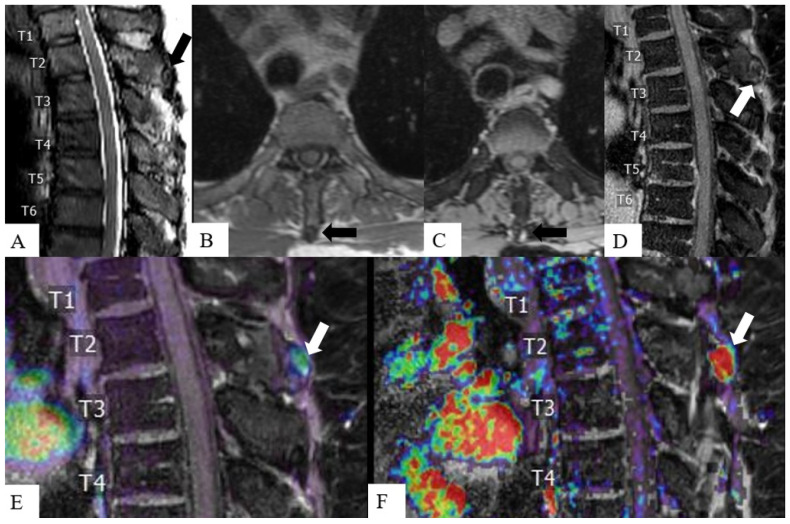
Metastatic lesion in the spinous process of the T2 vertebra. The sagittal T2-weighted image (A) displays a subtle oval-shaped lesion (arrow) involving the tip of the T2 spinous process. On pre-contrast axial T1-weighted image (B), the lesion shows low signal (black arrow), and peripheral enhancement on axial (black arrow) (C) and sagittal (white arrow) (D) post-contrast T1-weighted images. Post-contrast T1-weighted images overlaid with dynamic contrast-enhanced parametric maps improve lesion visibility and detection compared to conventional MRI, showing increased Vp (E) and K-trans (F) at the lesion site (white arrows).
